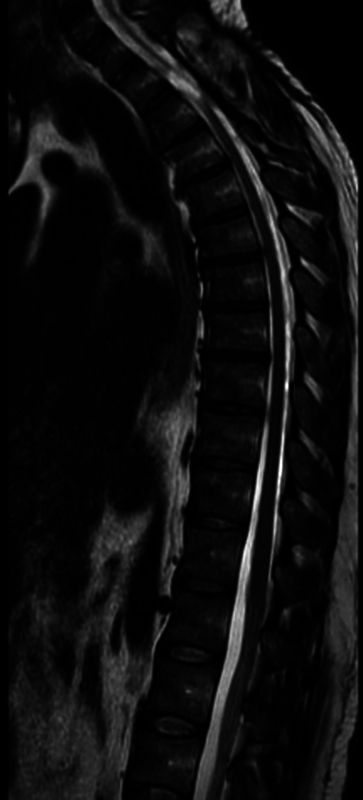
Post-surgical MRI of the spine– sagittal T2.
